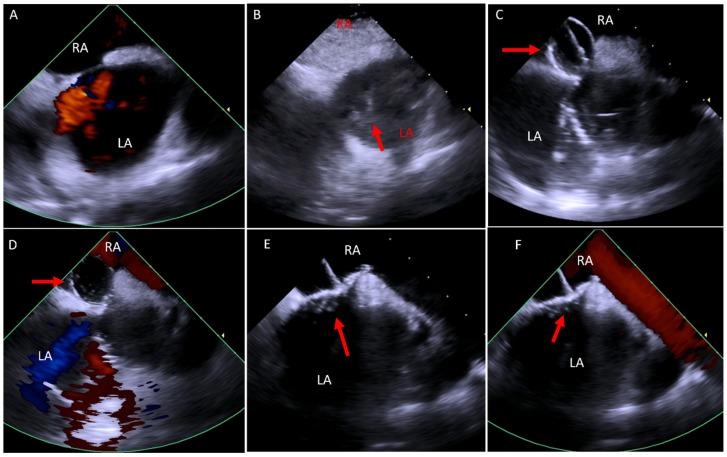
ICE catheter in the right atrium. (A) Color Doppler on the interatrial septum showing no evidence of shunt. (B) Agitated saline injection in the femoral vein with Valsalva maneuver showing positive study with bubbles crossing to the left atrium (red arrow) and confirming the diagnosis of PFO. (C,D) Balloon sizing and occlusion of the PFO without (C) and with (D) color Doppler, showing no evidence of further defects in the septum. (E,F) Deployment of PFO occluder (red arrow) without (E) and with (F) color Doppler and showing no residual shunt. ICE, intracardiac echo; LA, left atrium; PFO, patent foramen ovale; RA, right atrium.
